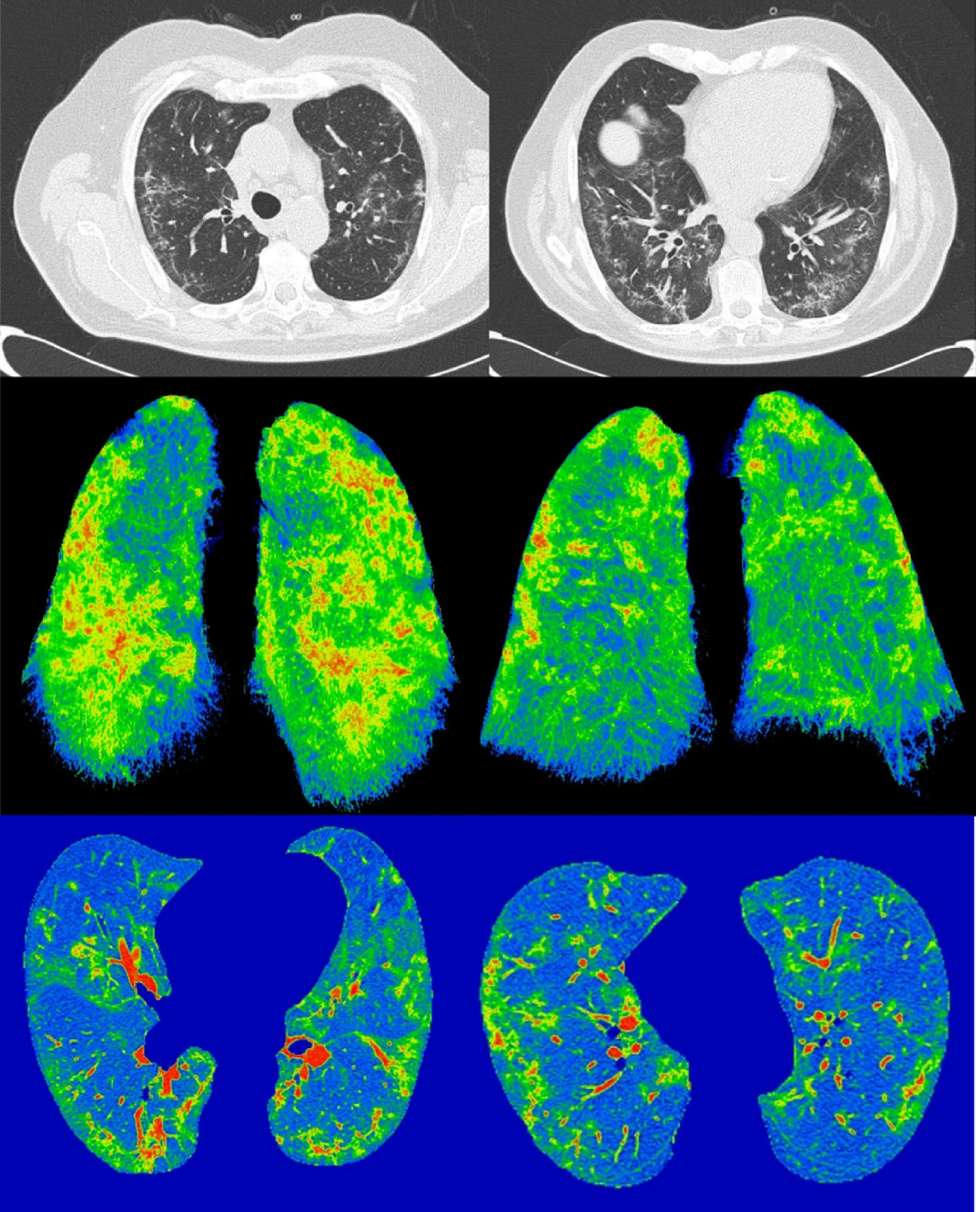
Example of lung volumes calculated by automatic segmentation tool on CT images by pre-setting a threshold value of Hounsfield Units and a color in order to obtain a segmentation of both lungs and a quantitative evaluation of emphysema (− 1024/− 977; blue), healthy residual lung parenchyma (− 977/− 703; yellow), GGO (− 703/− 368; pink) and consolidation (− 100/5; red)
